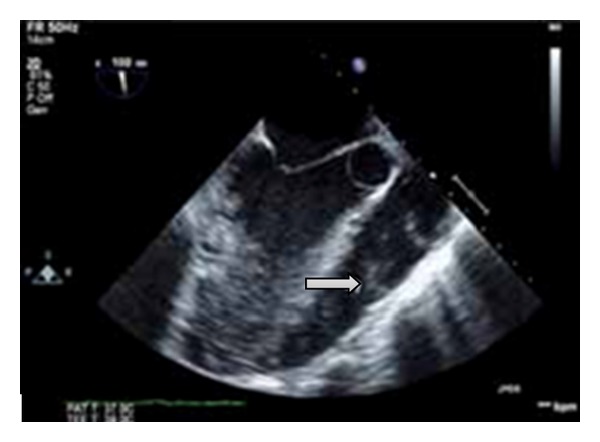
Echocardiogram shows a mass on the RVOT with association to the pulmonic valve (arrow).
